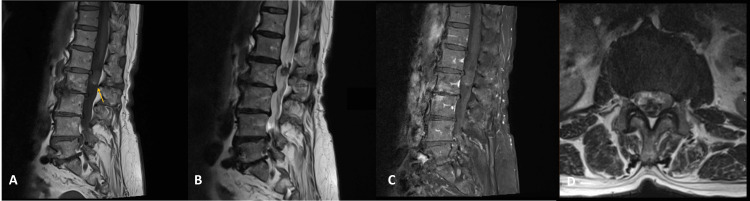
Cauda equina cavernoma on lumbosacral MRI sequences (A) Sagittal T1: An iso-/hyperintense nodular lesion at the L2 level. Arrow: caudal hyperintensity; (B) sagittal T2: hipointense nodular lesion; (C) sagittal post-contrast T1-weighted: almost no contrast enhancement; (D) axial T2
